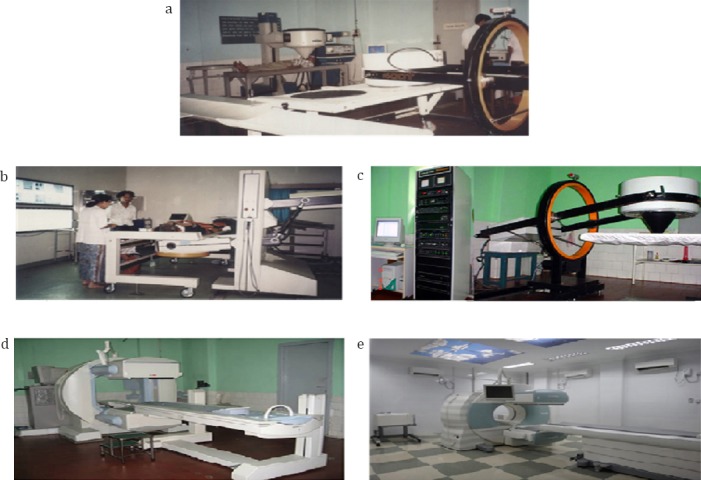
Gamma camera systems. a: 12.6.1985 Gamma muvek MB 9100 Gamma Camera, b: 18.2.1987 Hitachi Gamma Camera RC 135 DT, c: 24.5.1996 GE 400T Gamma Camera, d: 30.5.2003 SPECT Camera E Cam, e: 7.3.2014 SPECT CT Symbia
